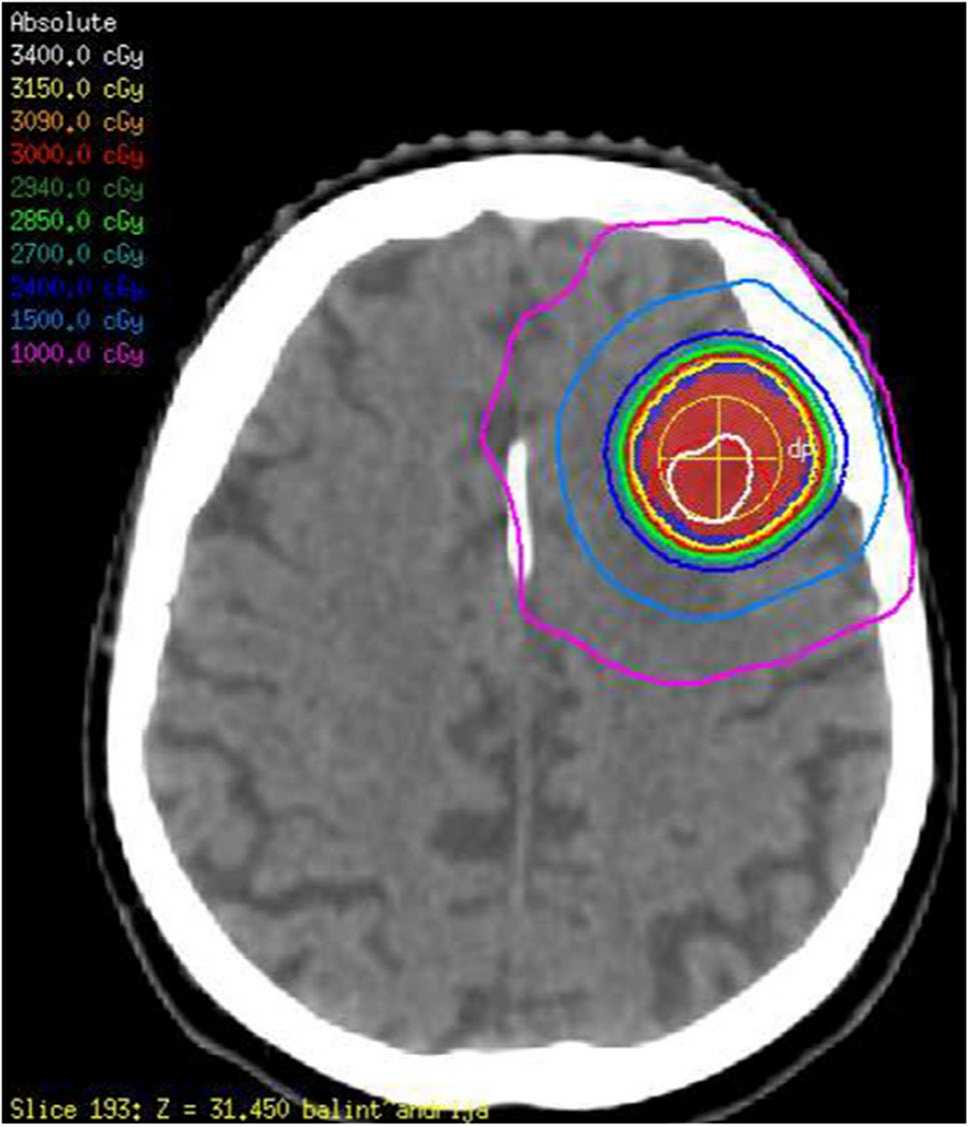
Treatment planning CT with the radiation isodose lines for a representative patient treated with 30 Gy in five fractions to a 2.7 cm frontal metastasis.
